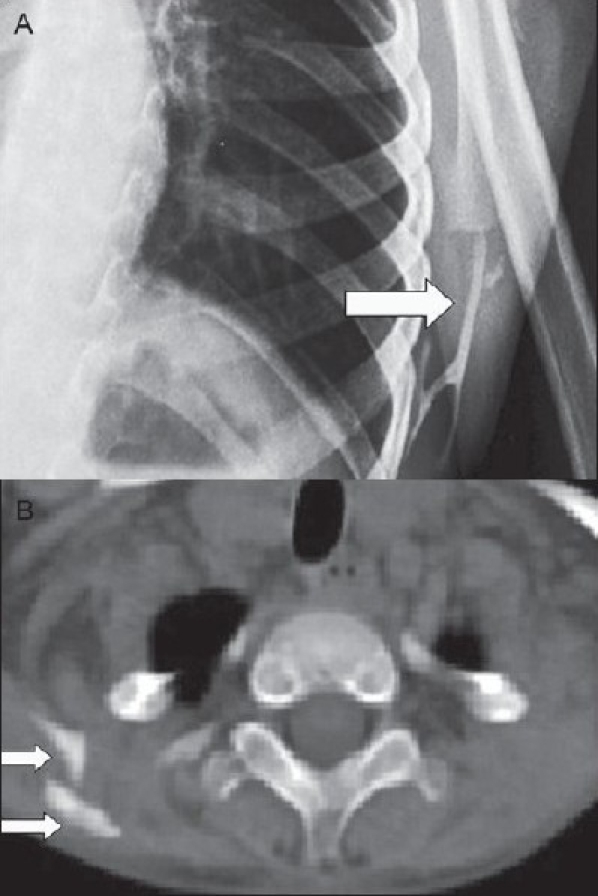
(A) X-ray chest showing calcific strands (arrow) around the inferior angle of the scapula. (B) CT thorax showing multiple plaque-like calcifications (arrows) in the left thoracic wall and paraspinal muscles
